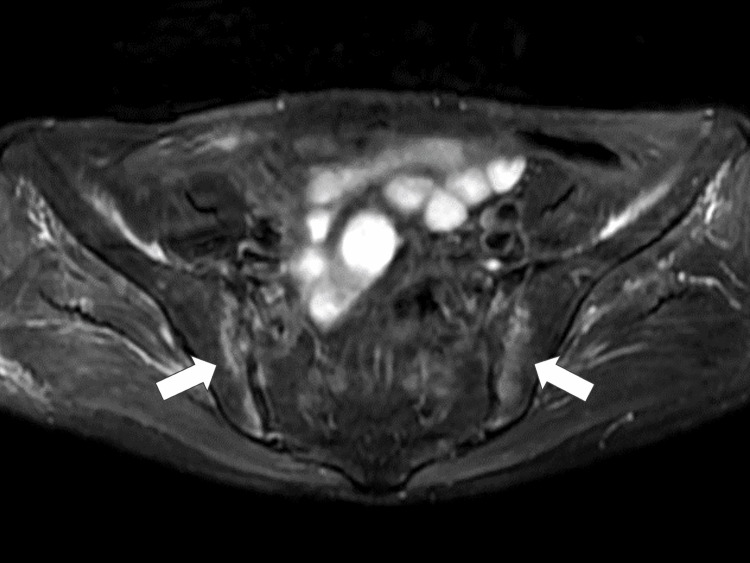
Pelvic magnetic resonance imaging scan revealing high signal intensity on short-tau inversion recovery in the bilateral sacroiliac joints (white arrows)
